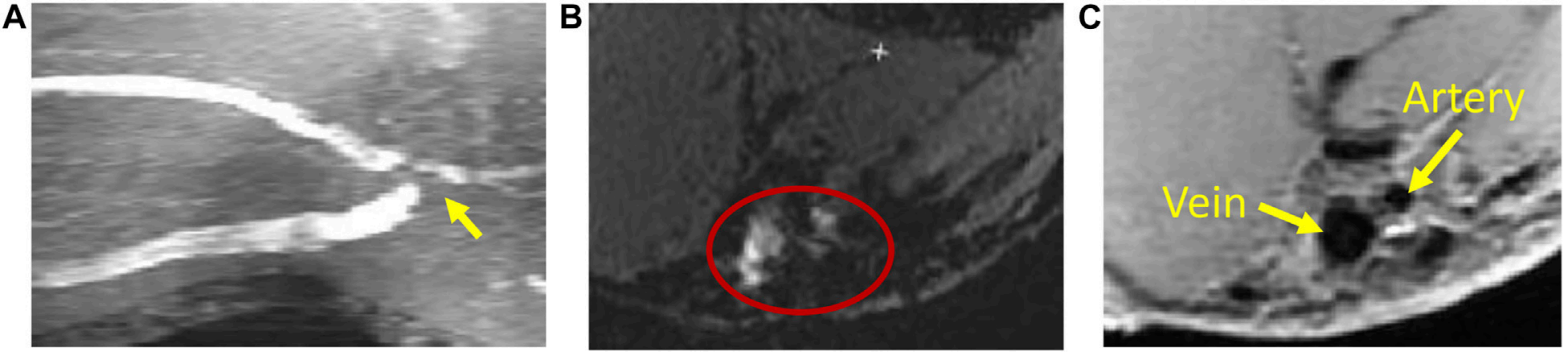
Magnetic resonance imaging of an arteriovenous fistula. (A) Maximal intensity projection of white-blood time-of-flight (TOF) images. At the anastomosis (the gray region pointed by a yellow arrow), the signal is void due to complex flow. (B) An example of the TOF slice close to the anastomosis (enclosed by the red ellipse) where the lumen is not clearly defined. (C) The black-blood image shows the lumen of the fistula vein and artery clearly (the black regions pointed by yellow arrows).
